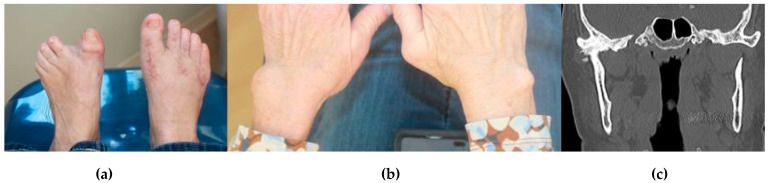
A 56-year-old female presented for evaluation with chief complaints of right TMJ pain and limited mandibular opening. Her history was most notable for long-standing RA refractory to multiple medications. (a) Bilateral toe involvement requiring Hoffman procedure; (b) Bilateral wrist involvement; (c) CT of the face showed early unilateral right TMJ ankylosis, lateral pannus formation, and heterotopic bone formation. The left TMJ was completely normal.
